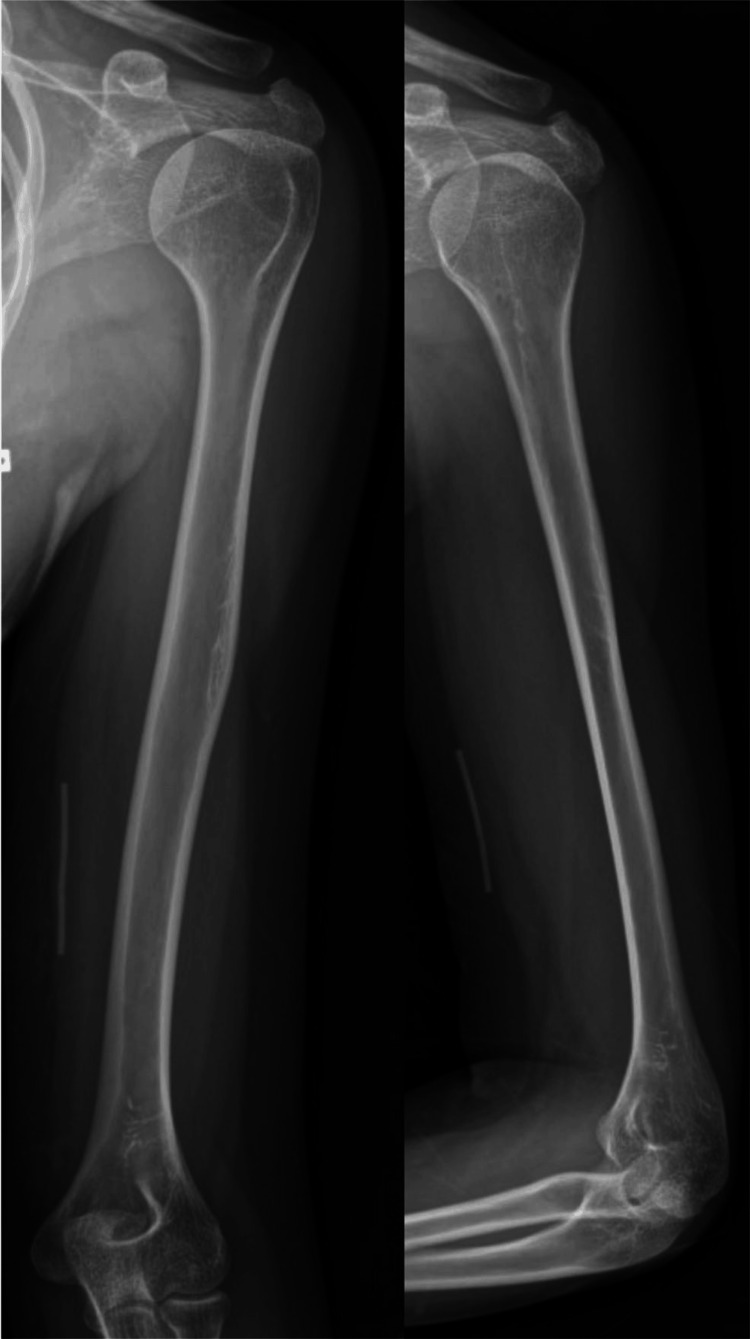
Radiograph of left humerus (anteroposterior and lateral views) showing the retained Implanon over the medial humerus
